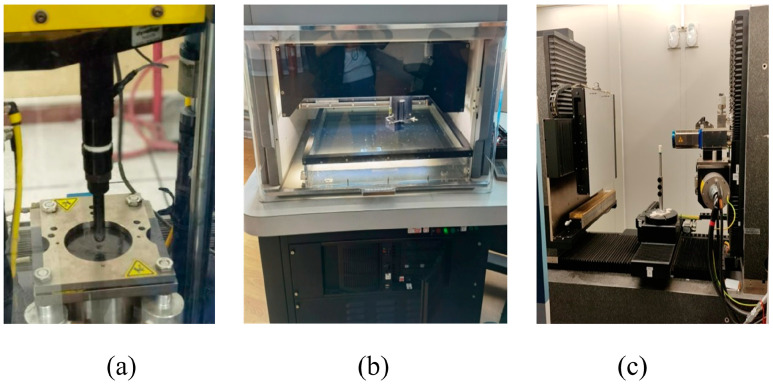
Test equipment and inspection equipment: (a) Drop weight impact test bench; (b) Ultrasonic testing system; (c) X-ray testing device.
